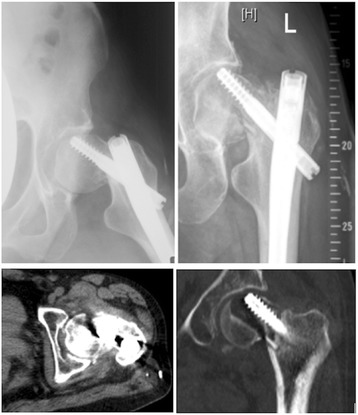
Follow-up radiographs (5 months post-op) and CT scan (7 months post-op) of pelvis and left hip, demonstrating lag-screw cut-out with displacement of the femoral neck fracture
